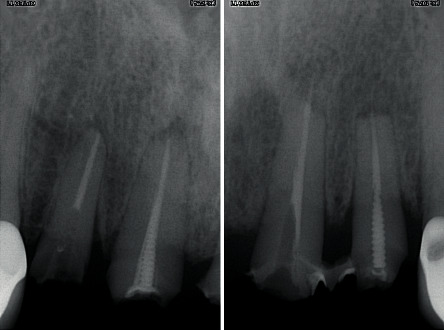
Periapical X-rays of the 4 incisive roots.
